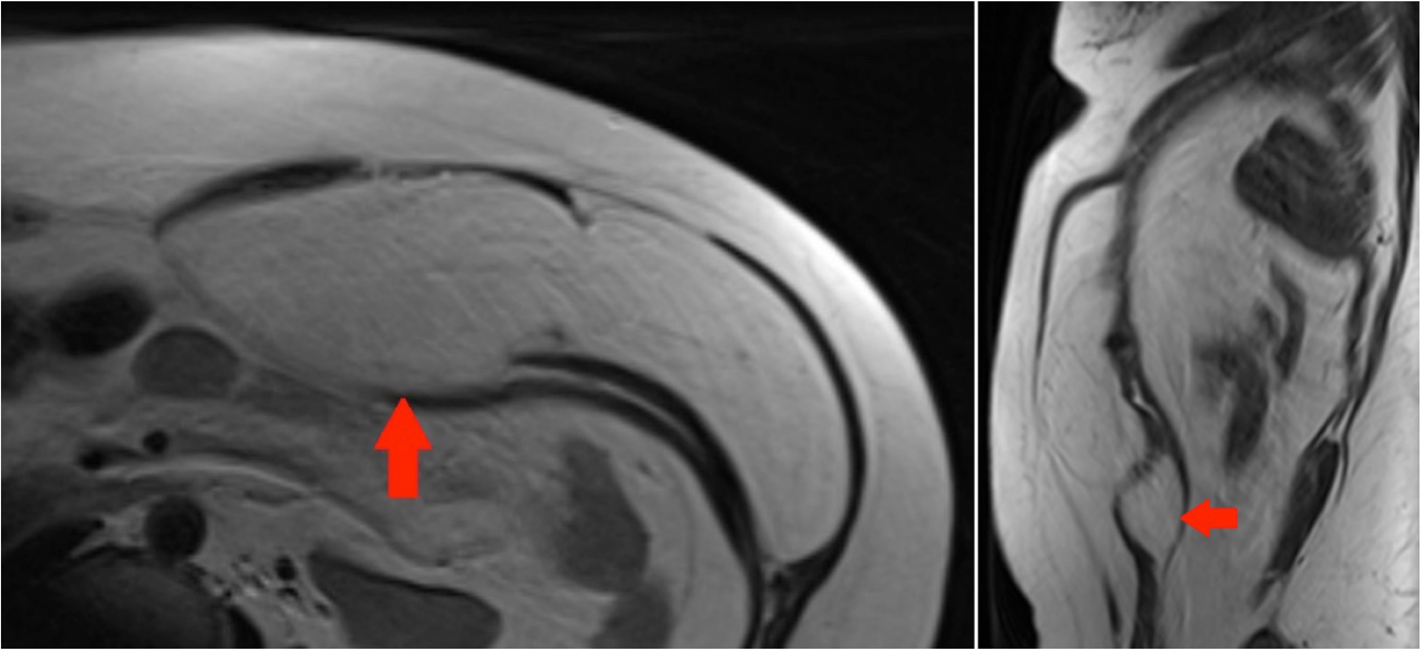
Left panel: Axial T1-weighted image of the abdomen: fatty lesion deep to the left rectus (red arrow) extends laterally under the external oblique muscle. Right panel: Sagittal T1-weighted MR image of the abdomen: interparietal hernia splitting the lateral abdominal wall muscles (red arrow)
